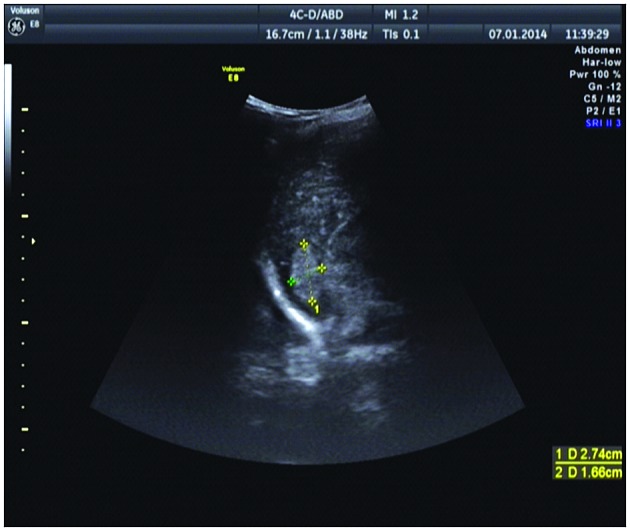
B-ultrasonic examination revealing a mass in the retroperitoneal region.
